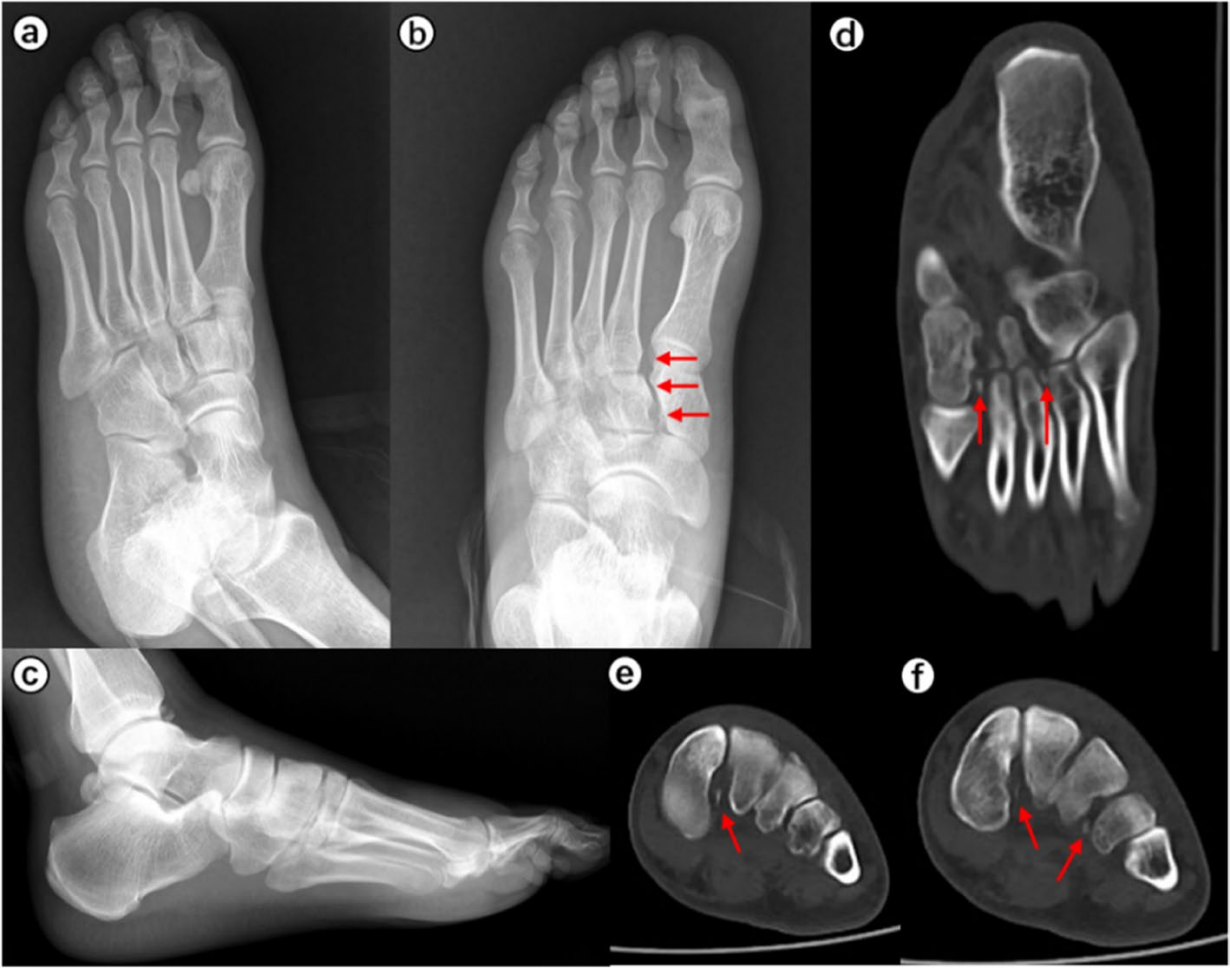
A 37-year-old man fell and hurt his left foot. Chiodo-Myerson’s classification: medial–lateral-column injury; Displacement classification: displaced injury. Both two observers made the correct diagnosis for two times, and didn’t change the initial treatment option (surgery) after evaluating CT image. a-c The conventional radiographs showed no obvious fractures. The separation of the first and second rays strongly suggested Lisfranc injury (red arrows). d-f Computed tomography imaging showed intra-articular avulsion fractures of the medial cuneiform and fourth metatarsal bones. Red arrows indicate the fracture fragments
